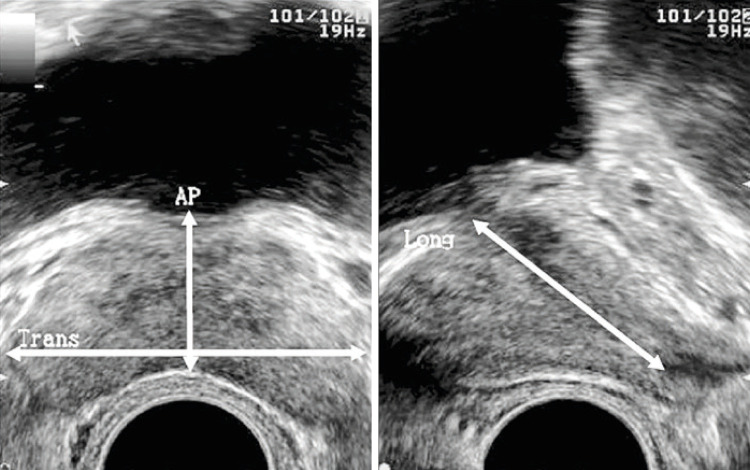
Volume prostático por ultrassonografia transretal
